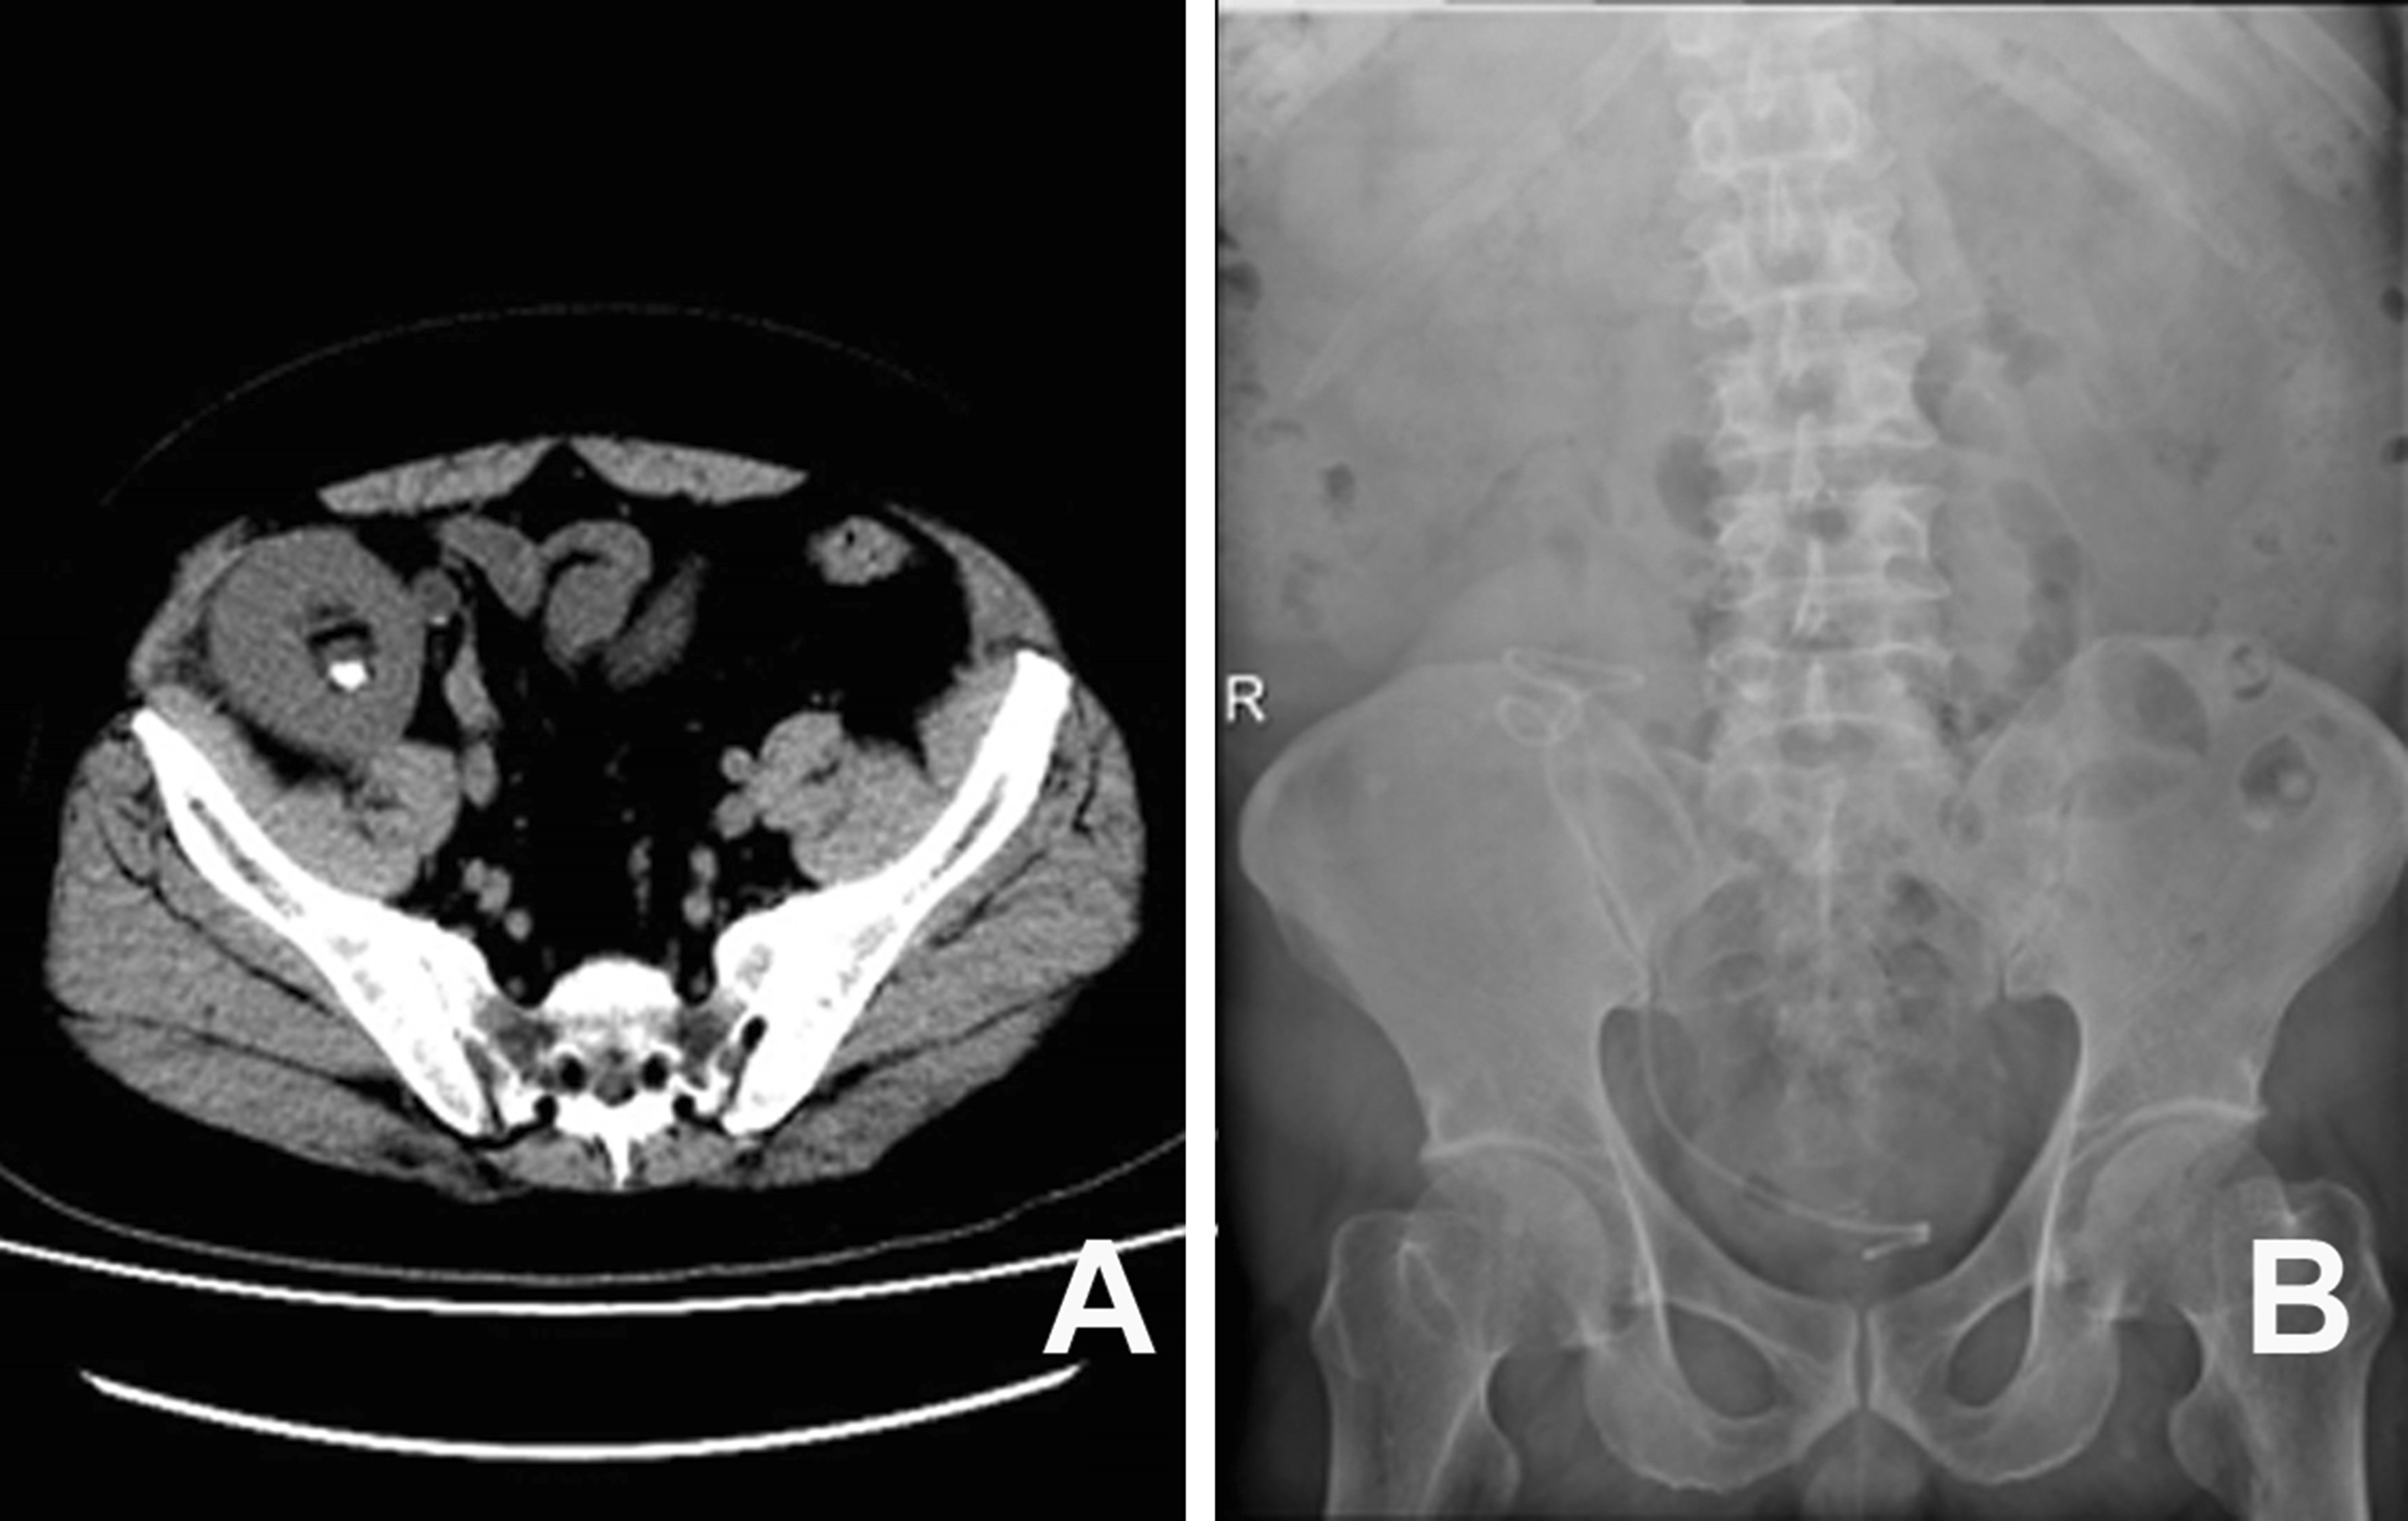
The placement of a double-J stent in a 27-year-old male developing ureterovesical junction stricture and ureteral calculi. a The computed tomography revealed multiple transplant ureteral calculi. b The KUB film revealed that the double-J stent was inserted into the pelvis
